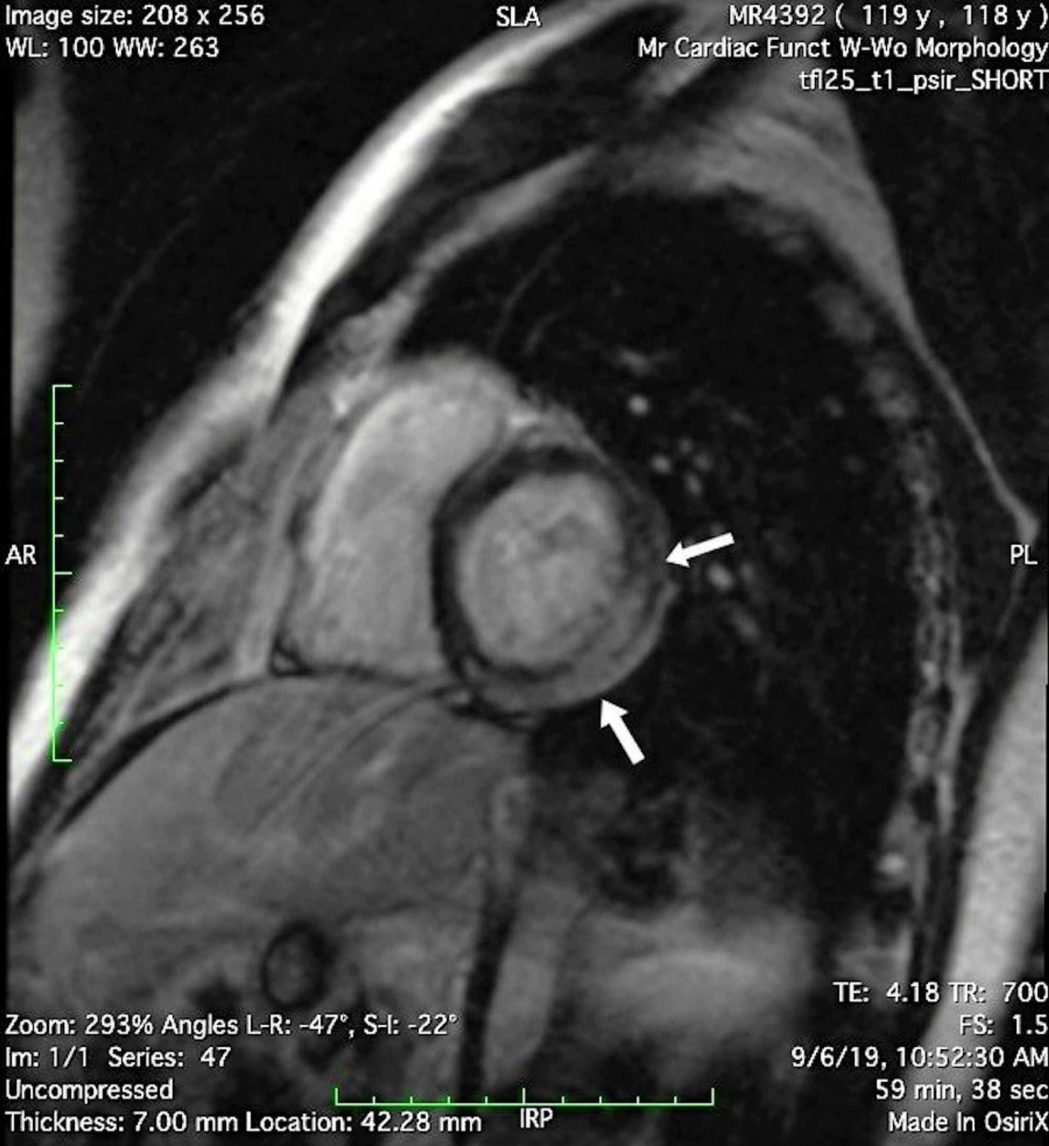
CMRI of the patient The arrows show delayed enhancement of the midmyocardium, epicardium, and adjacent pericardium at the mid-cavity level of the left ventricle. This has been interpreted as extensive myocarditis/pericarditis in the inferior and lateral wall of the left ventricle CMRI: cardiac magnetic resonance imaging
